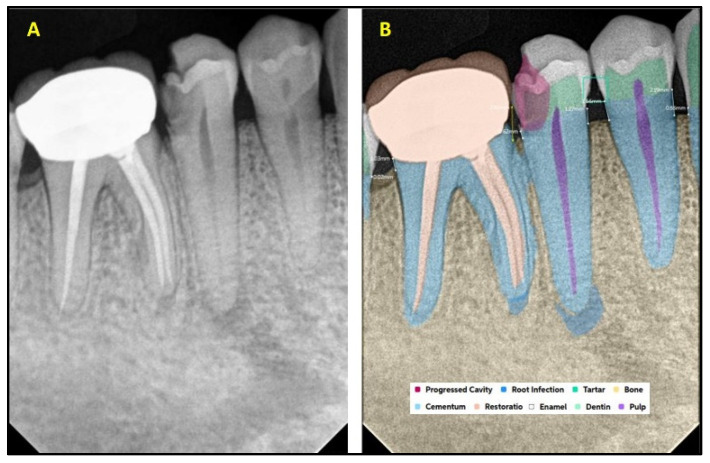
(A) Intraoral periapical radiographic image of mandibular posterior teeth; (B) analysis made by artificial intelligence software depicting diagnosed conditions (dental caries, crown, bone loss, periapical lesion, endodontic treatment, and calculus).
